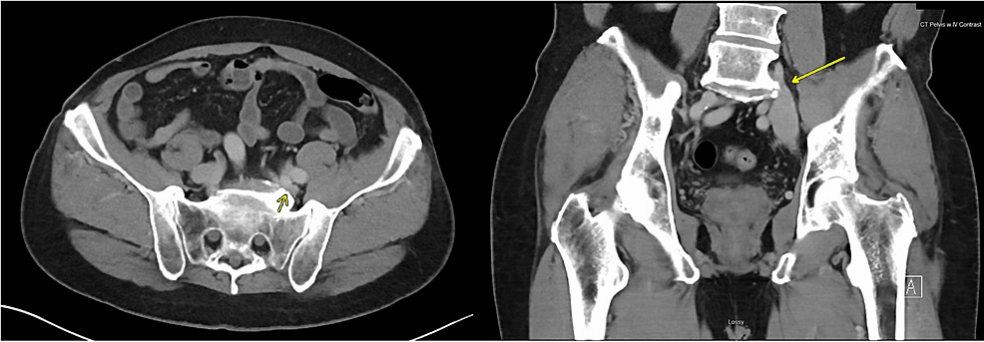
Left common iliac vein compression CT scan of pelvis with contrast showing extrinsic compression of the left common iliac vein between the left internal iliac artery and fifth lumbar spine (yellow arrows)
